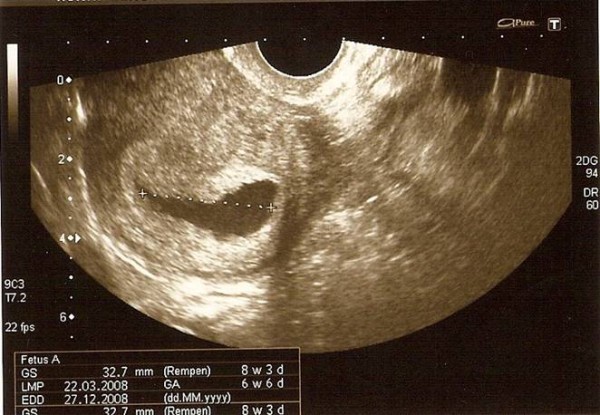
Transvaginal ultrasound of uterus showing an irregular intrauterine gestational sac of approximately 8 weeks.
